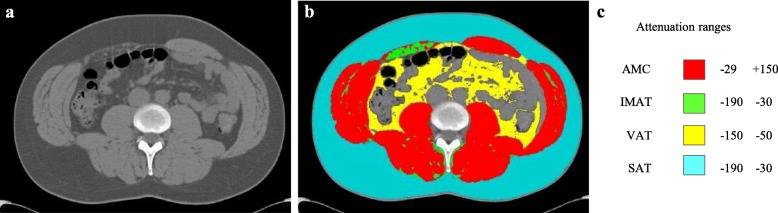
Example of segmentation. Original computed tomography image (a). Segmented image (b). Attenuation ranges defined for each segmented tissue (c)
